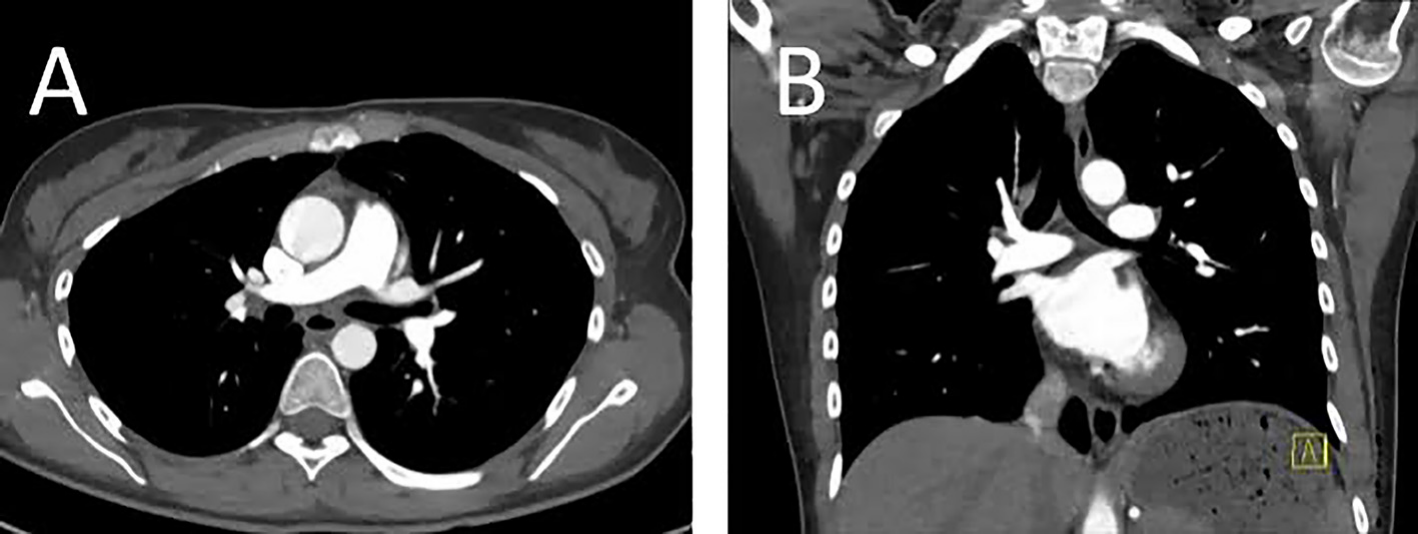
High-pitch CT pulmonary angiogram shown as transverse (A) and coronal (B) reconstructions in a 33-year old lean female patient (BMI 21, effective chest diameter 23 cm) with no evidence of pulmonary embolism. Image quality was rated as "excellent" by both readers.
